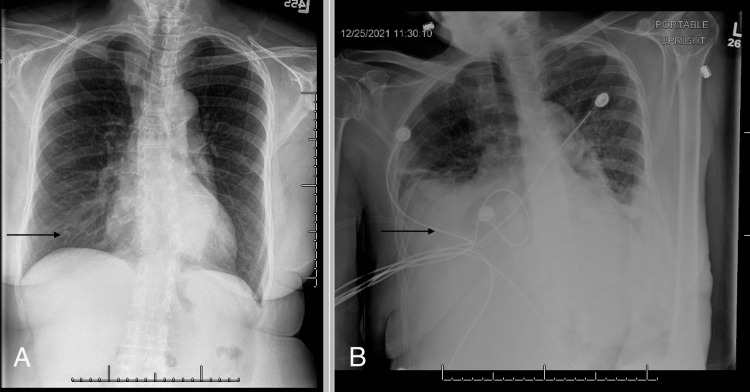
Comparison of chest X-ray between 2017 and 2021. This figure compares a chest radiograph in 2017 (A) to one in 2021 (B). The prior X-ray (A) was negative for pathological processes. The newer X-ray (B) shows a pleural effusion consistent with a large mass (see arrow).
